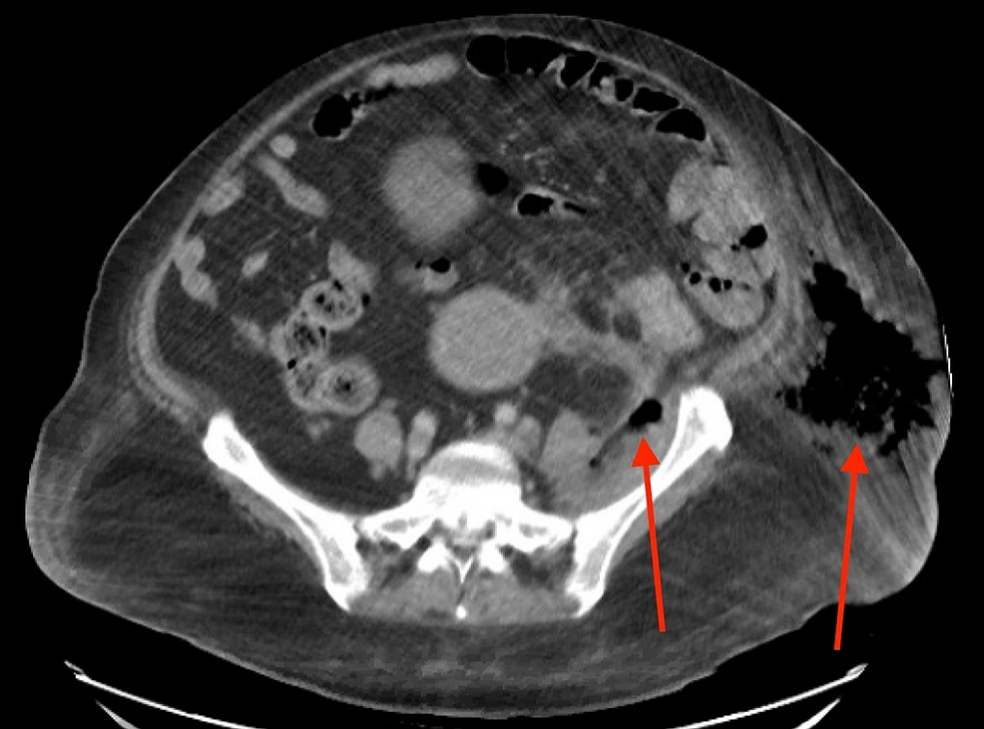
CT abdomen and pelvis showing subcutaneous air in the retroperitoneum extending into the left flank
